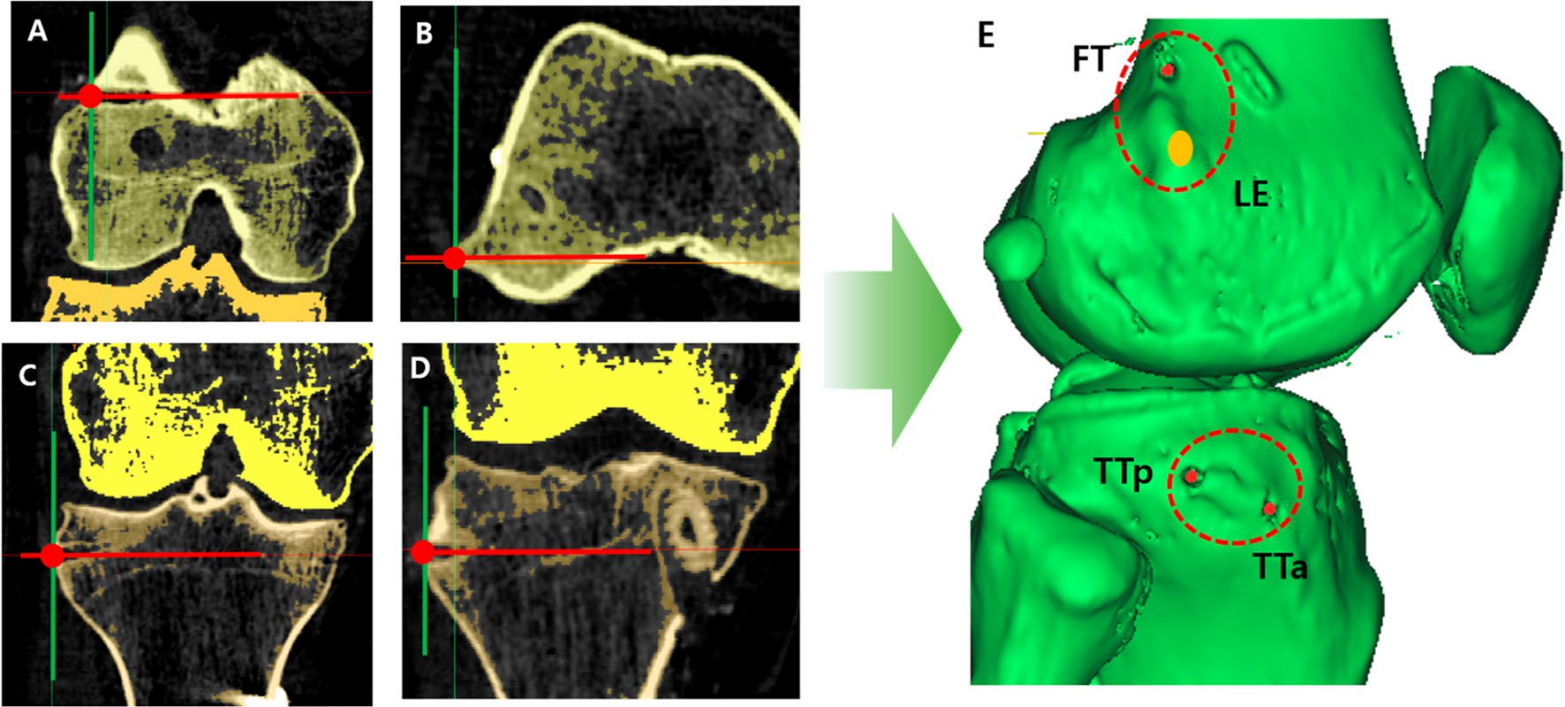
3D CT reconstruction and setting of the center points of each landmark. The center point (red circle) at the A femoral tunnel (FT), B lateral epicondyle (LE) of the femur, C posterior tunnel of the tibia (TTp), D anterior tunnel of the tibia (TTa), and E 3D-reconstructed image developed by setting the center point of the LE (yellow circle)
